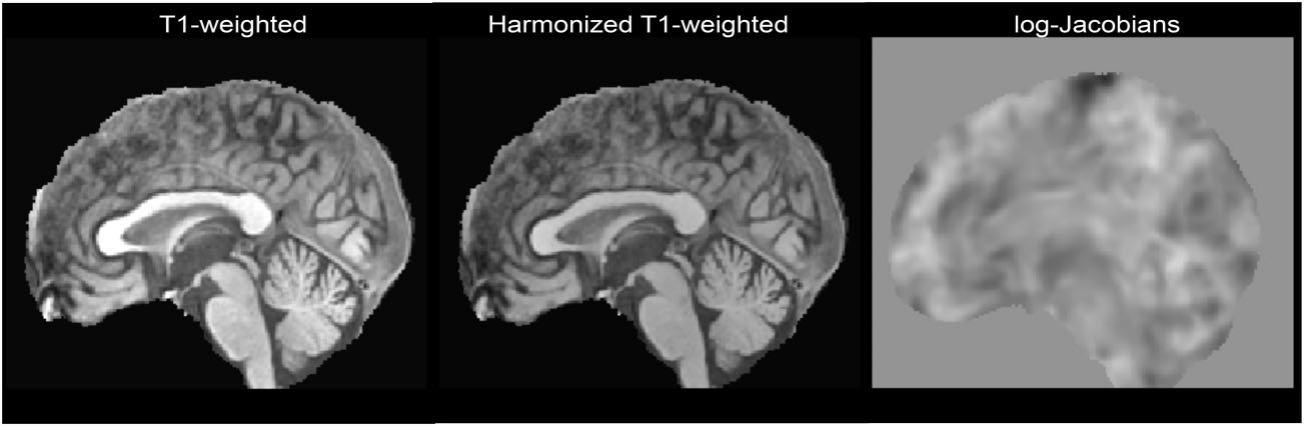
Example imaging data. T1-weighted denotes the preprocessed raw MRI brain scans, harmonized T1-weighted denotes the T1-weighted MRI scans after intensity harmonization (histogram matching), and log-Jacobians denotes the deformation fields generated during registration.
